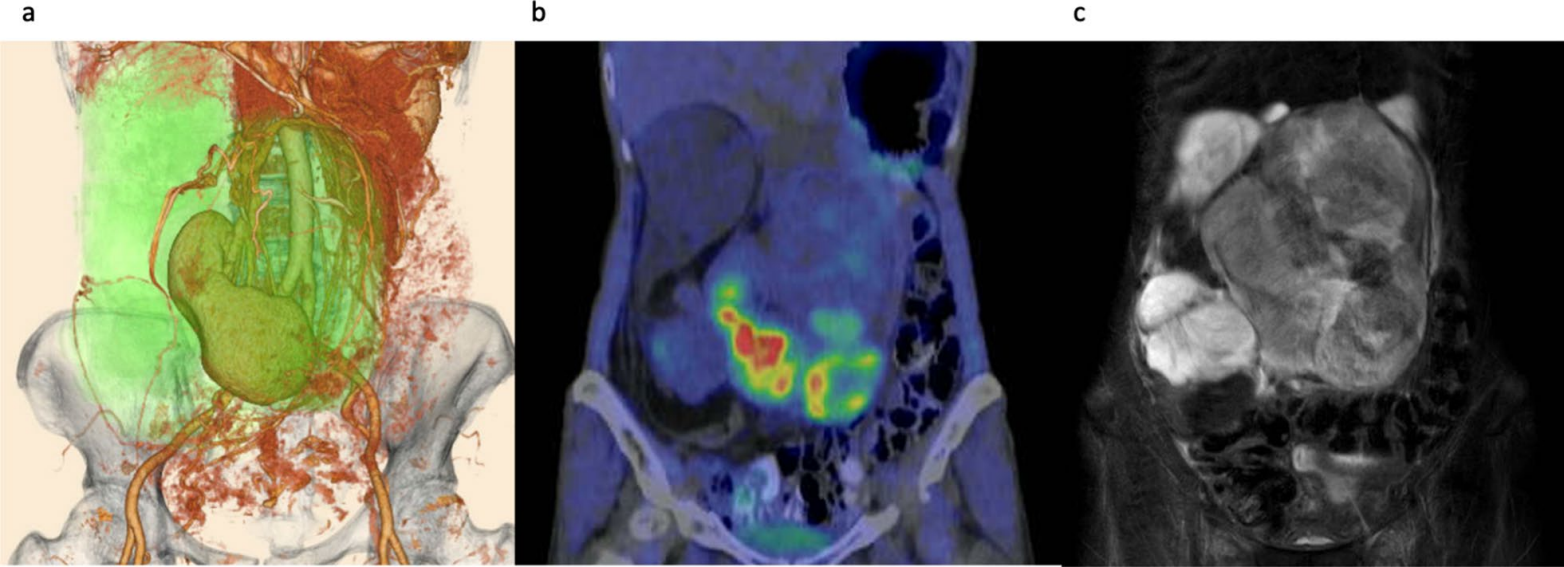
Preoperative image. Three-dimensionl image of computed tomography reconstruction revealed retroperitoneal huge tumor surrounding right kidney (green area) (a). Positron emission tomography–CT showed a mixture of areas with and without fluorodeoxyglucose accumulation (b). T2-weighted magnetic resonance imaging showed a mixture of fatty and myxoid components (c)
